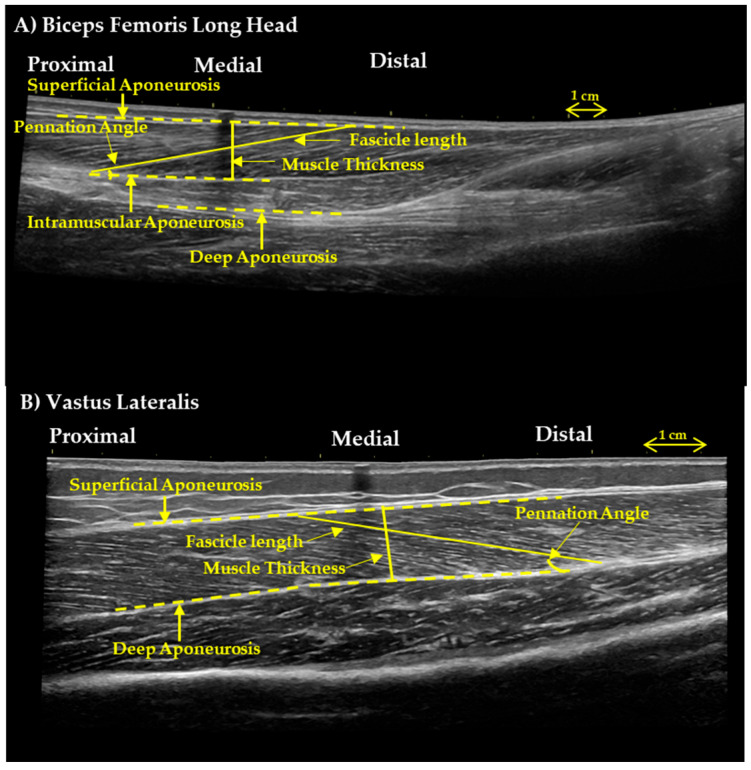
Panoramic ultrasound images of (A) biceps femoris long head and (B) vastus lateralis.
